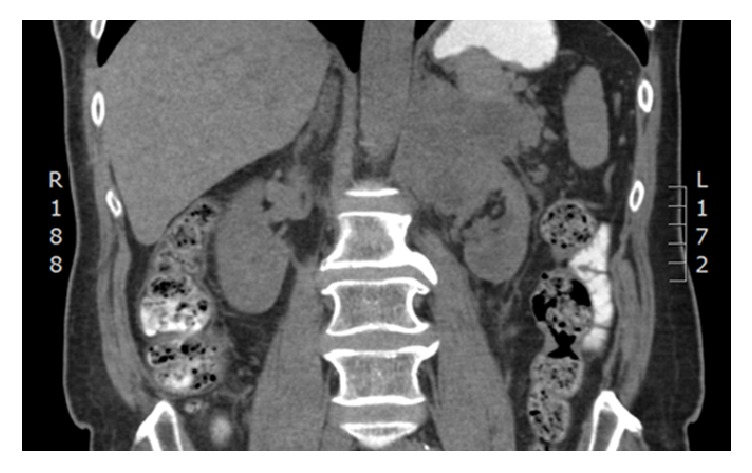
CT abdomen coronal view showing a large cystic left renal mass with suspected splenic hilar invasion.
